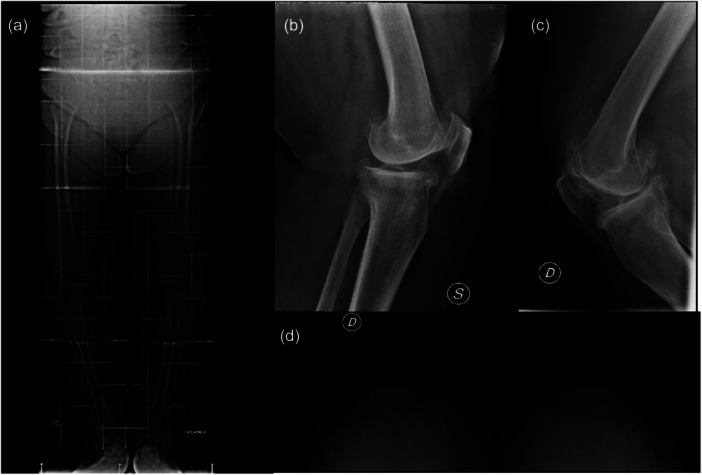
Preoperative full‐length standing radiograph of the lower limbs (a), lateral (b, c) and axial patellar (d) views of a bilateral case.
